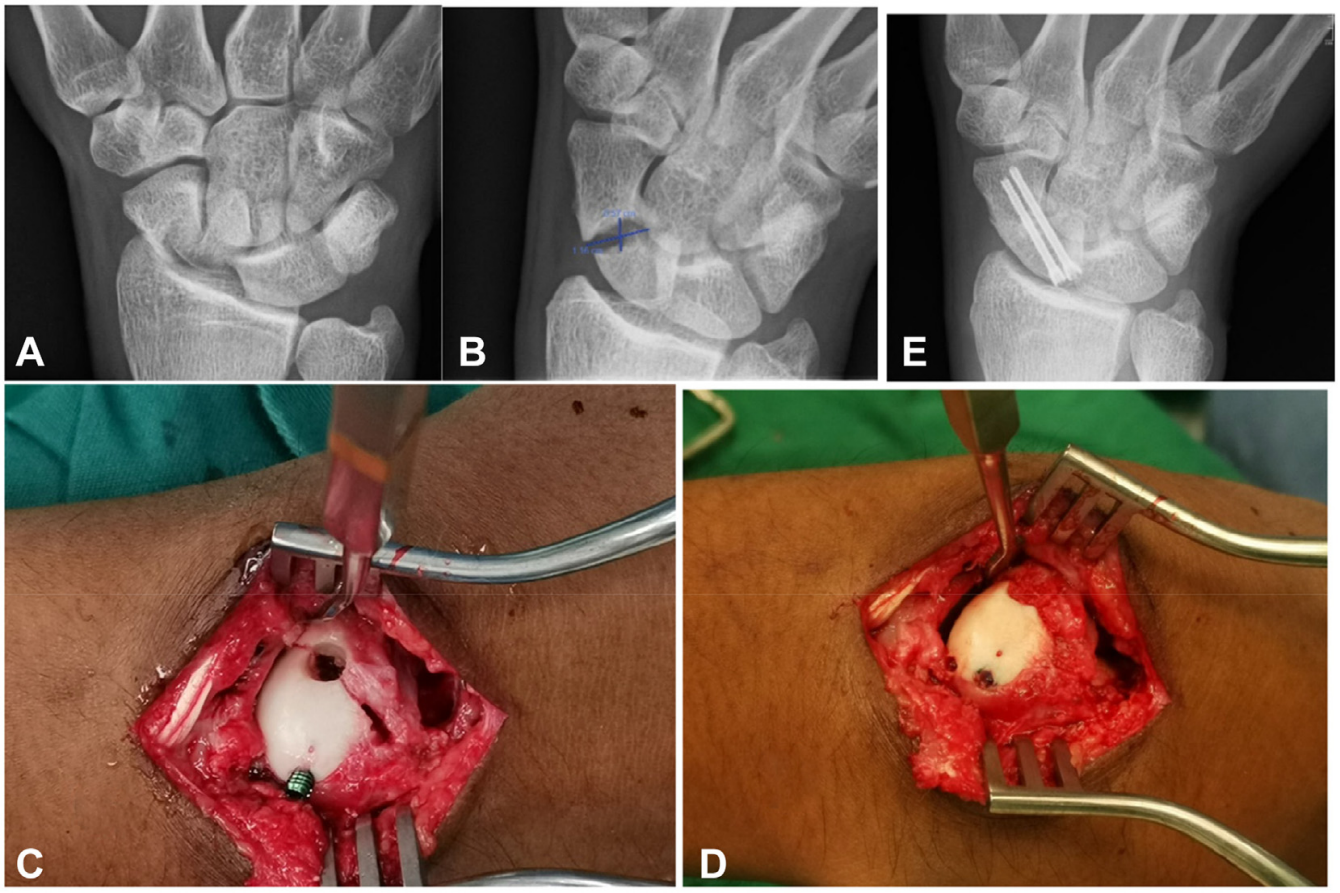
Case no 4. Waist fracture with unstable and widely displace fragment for “Perforate and Fill” bone grafting technique. (A) Radiograph showing widely displaced unstable fracture nonunion. (B) Radiograph showing fracture cavitation of L 11.6 mm × H 5.7 mm. (C) intraoperative picture showing reduction of the fracture, interim stabilization of the fracture with partial fixation, and the 4 mm burr holes to access the fracture cavity. (D) Showing the cavitation being filled with bone grafts and compacted and showing the final completion of the fixation.
